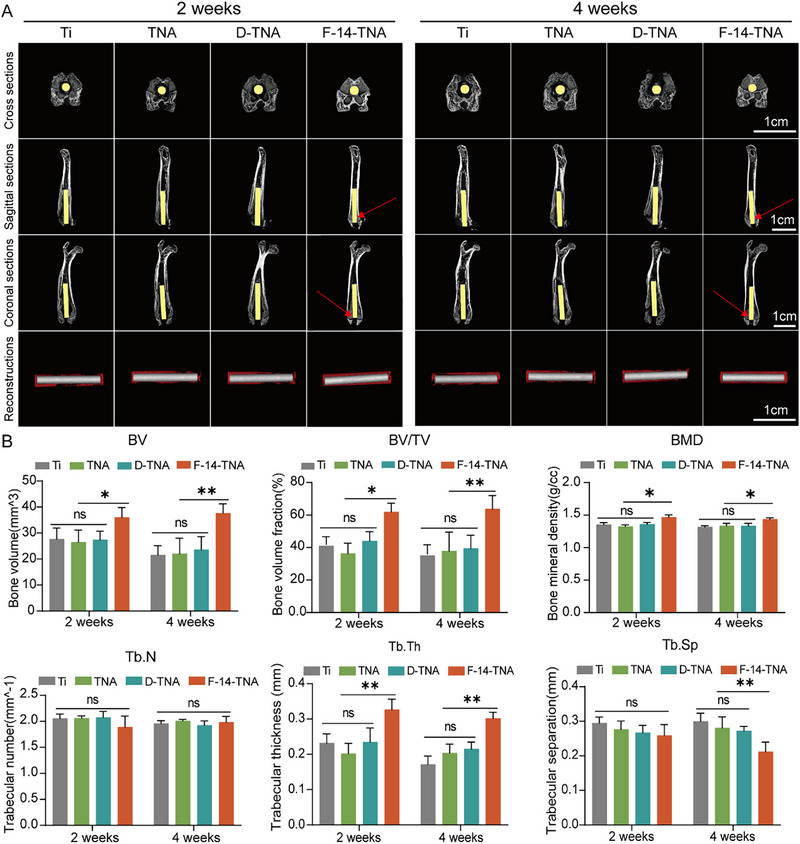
Micro‐CT assessment results. A) Cross sections, sagittal reconstructions, coronal sections, and reconstructions (yellow labels highlight the implant, red labels indicate bone tissue, and red right shows bone and implant interface integration region). B) Quantitative results of the BV, BV/TV, BMD, Tb. N, Tb. Th, and Tb. Sp in the region of the reconstructions. The data represent the mean ± SD; n = 6; p* < 0.05, *p* < 0.01, and ns: not significant.
